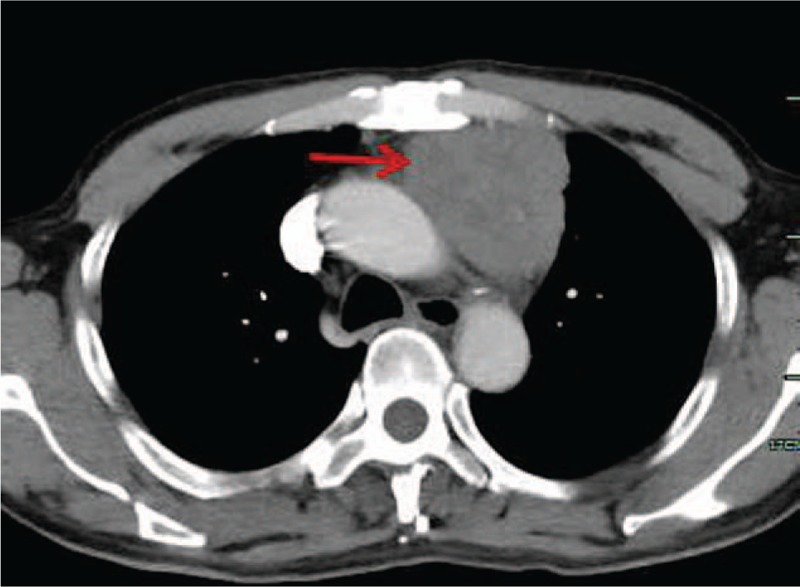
The contrast-enhanced CT revealed a 4.5 × 4.0 cm solid mass involving the anterior mediastinum (arrows).
